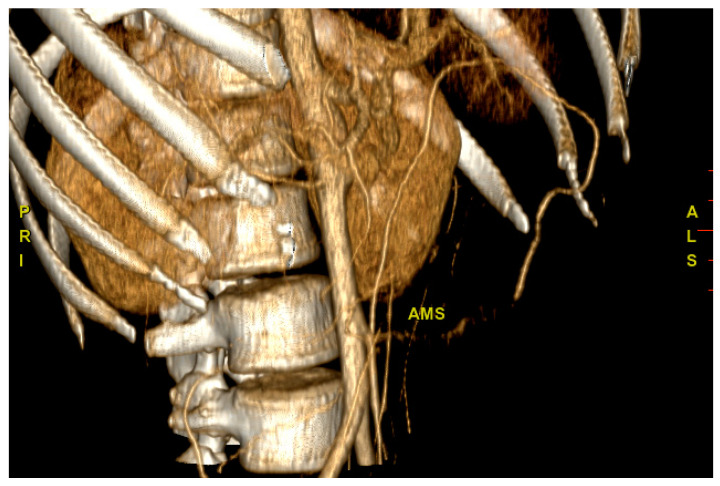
CT scan of the abdomen. Proximal occlusion of the superior mesenteric artery (AMS).
